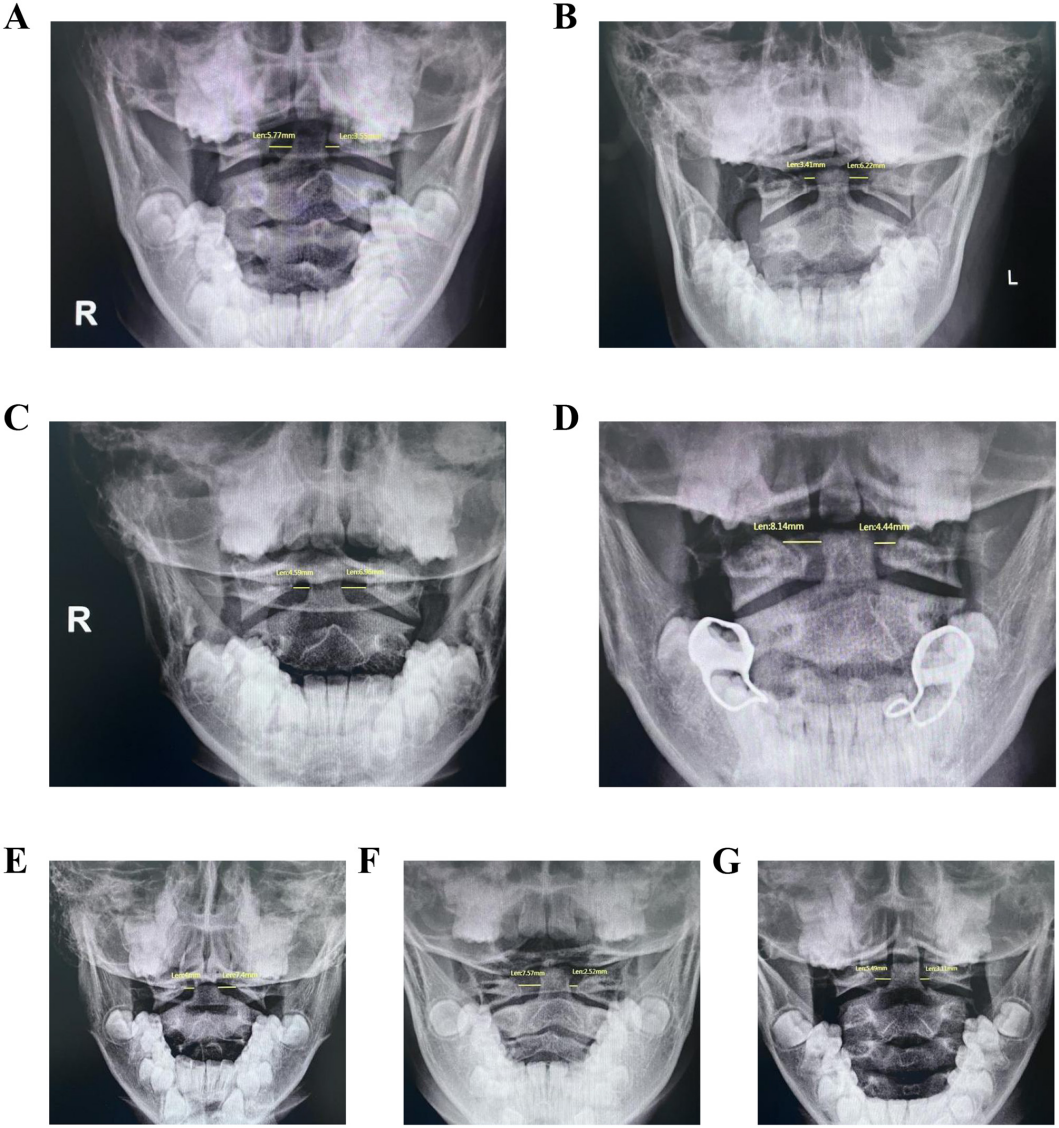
(A–G) The X-ray manifestations of the atlantoaxial opening position in children with TD of different ages and genders. The left side of the image corresponds to the right side of the human body, with “R” indicating right and “L” indicating left. The yellow lines represent the left and right LADI measurements (unit: mm). LADI is defined as the shortest distance from the lateral margin of the odontoid process (axis) to the medial margin of the lateral mass (atlas).
